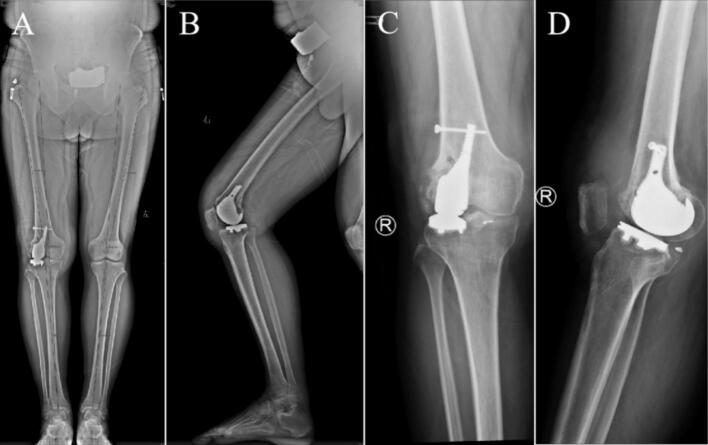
The X-ray images at eighth postoperative week. (A) Full-length AP view of both lower extremities. (B) Lateral view of the right lower extremity. (C) AP view of the right knee. (D) Lateral view of the right knee.
